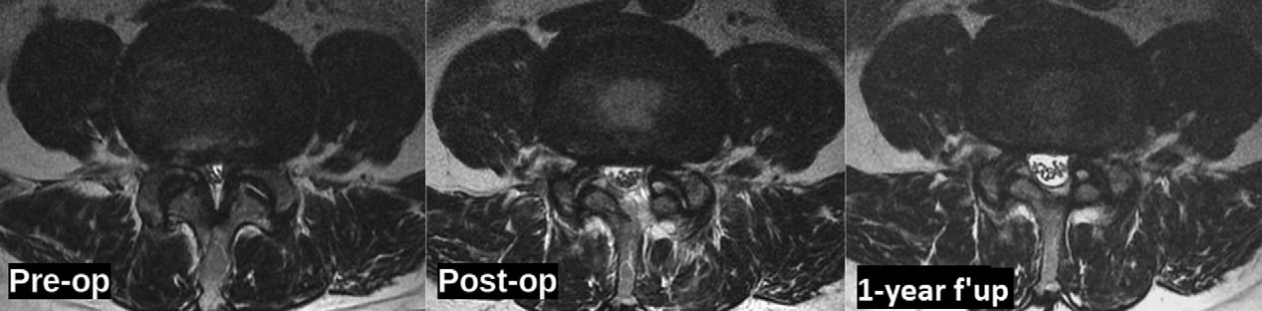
Case example of a 60yrs old female with L4-5 central canal stenosis with sacralized L5. The MRI scan axial cut done at preoperative, immediate postoperative, and 1-yr follow-up showing significant improvement in the lumbar canal cross sectional area at the operative level. MRI = magnetic resonance imaging.
